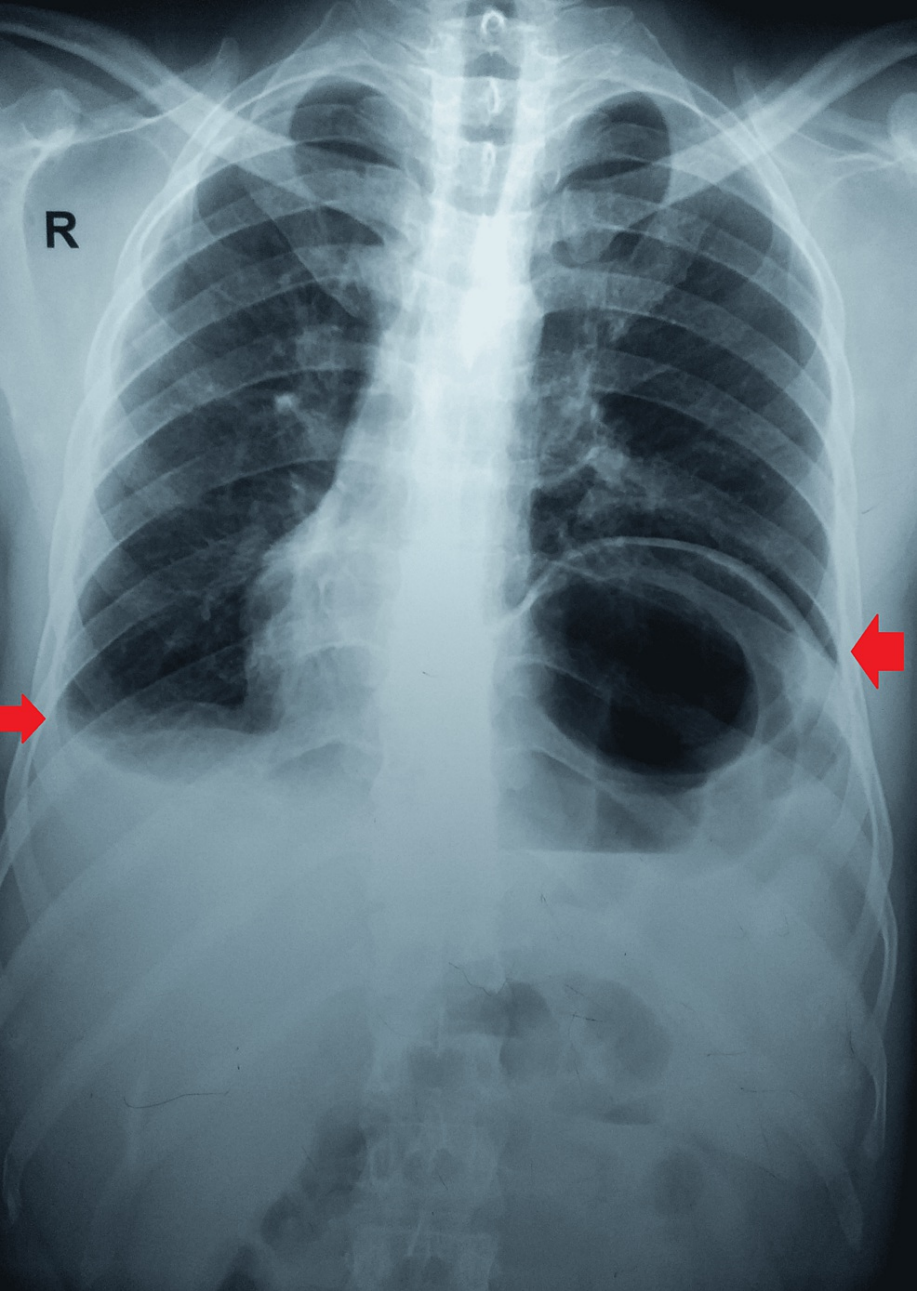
A plain chest radiograph suggestive of a raised dome of the left diaphragm pushing the heart to the right and blunting of the costophrenic angle on the right. Arrows suggestive of left diaphragmatic eventration and right pleural effusion.
